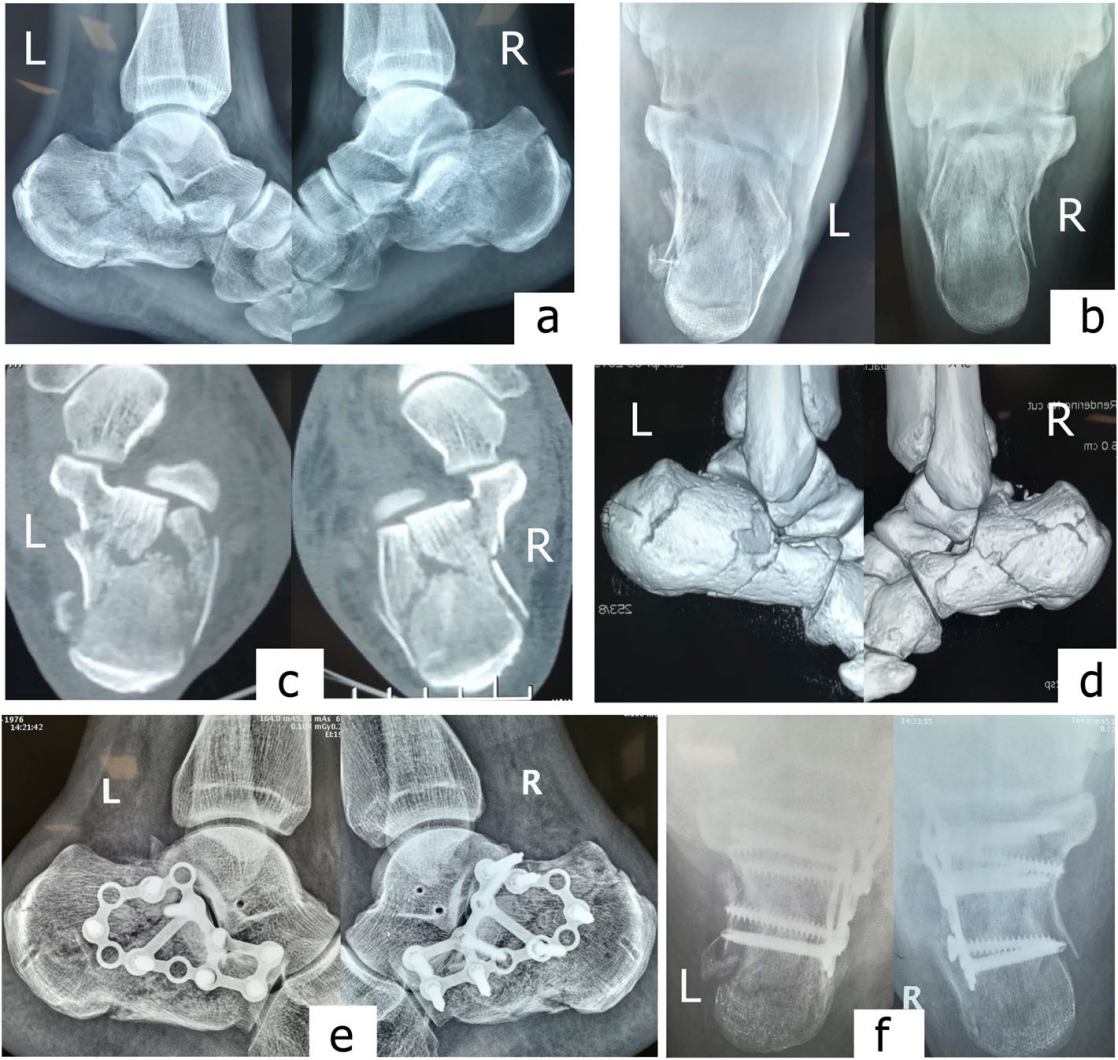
A 43-year-old male patient falls from a height of 4 m, over 10 years of smoking history. Preoperative lateral (a) and axial (b) radiograph showed bilateral calcaneus fracture with reduced Böhler angle (L: 2°, R: 5°), length (L: 80 mm, R: 75 mm), and height (L: 70 mm, R: 68 mm), and width (L: 44 mm, R: 42 mm). The preoperative horizontal computed tomography image (c) showed Sanders type IIIAC fracture. Steinmann pin retractor-assisted reduction with circle plate fixation via the sinus tarsi approach was used (d). Postoperative lateral (e) and axial (f) radiographs show obvious correction of the Böhler angle (L: 30°, R: 36°), and improvements in calcaneal length (L: 88.1 mm, R: 85 mm), height (L: 82.8 mm, R: 82.6 mm), and width (L: 36.2 mm, R: 36.9 mm). AOFAS score was 87 for left and 82 for right at the last follow-up
